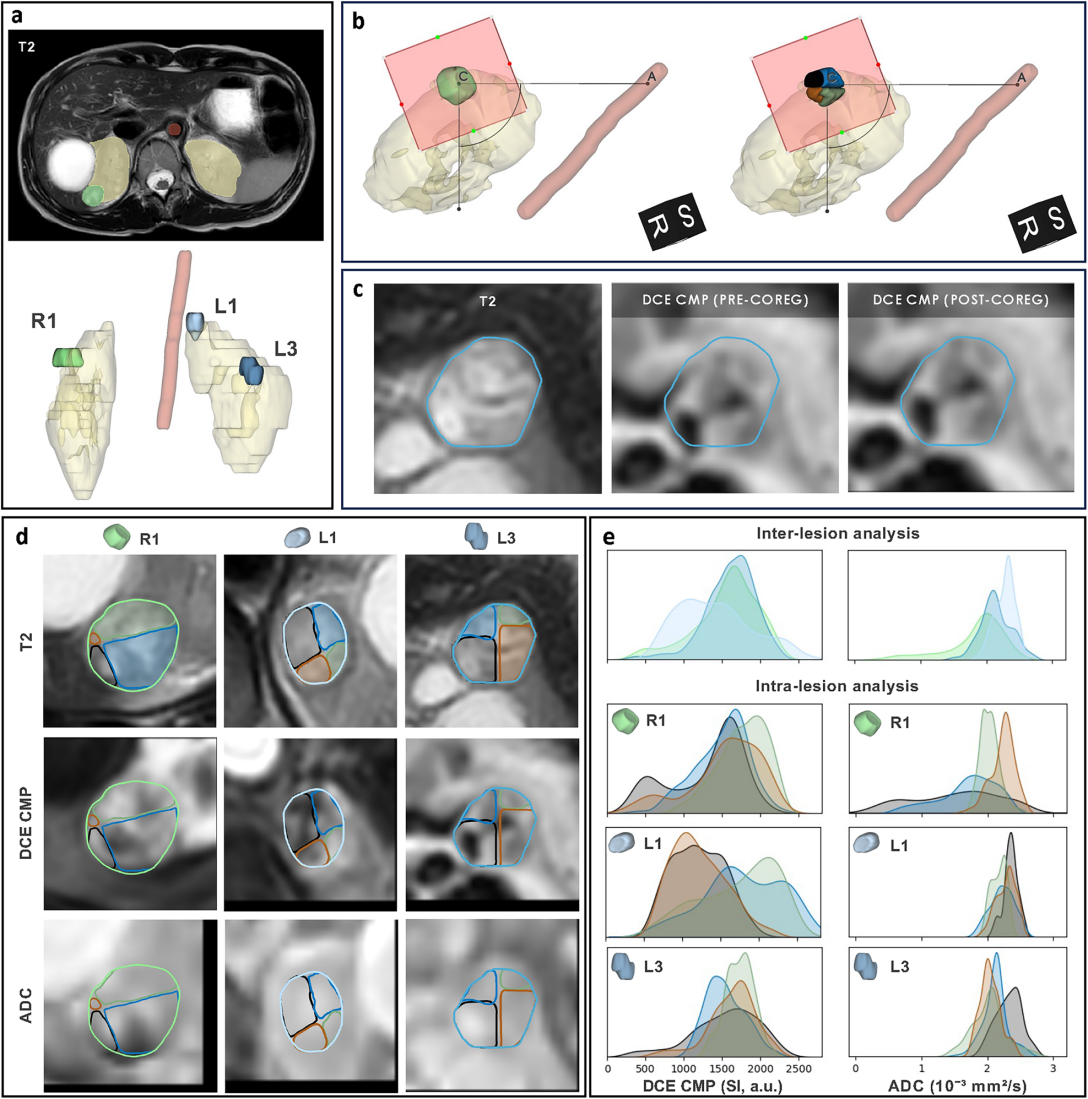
Postprocessing and multiparametric analysis of magnetic resonance images revealing intra- and inter-tumour heterogeneity. Postprocessing: (a) segmentation of left and right kidneys, lesions (R1, L1, and L3), and the descending aorta using T2W images; (b) definition of a lesion-specific reference system based on identifying the approximated resection plane (red square) and the axis connecting the lesion centre C to the descending aorta A, enabling the subdivision of each lesion into four quadrants; (c) lesion-specific multi-parametric three-dimensional patches, *e.g*., DCE corticomedullary phase (DCE CMP) of L3 lesion shown in the panel) are extracted and coregistered with the T2W patches. Multiparametric analysis: (d) whole-lesion and quadrant-specific contours are highlighted on axial views of T2W images, DCE CMP images, and ADC maps. e Kernel density estimate plots compare DCE CMP, and ADC value distributions between whole lesions R1, L1, and L3 (inter-lesion analysis) and between quadrants within each lesion (intra-lesion analysis)
